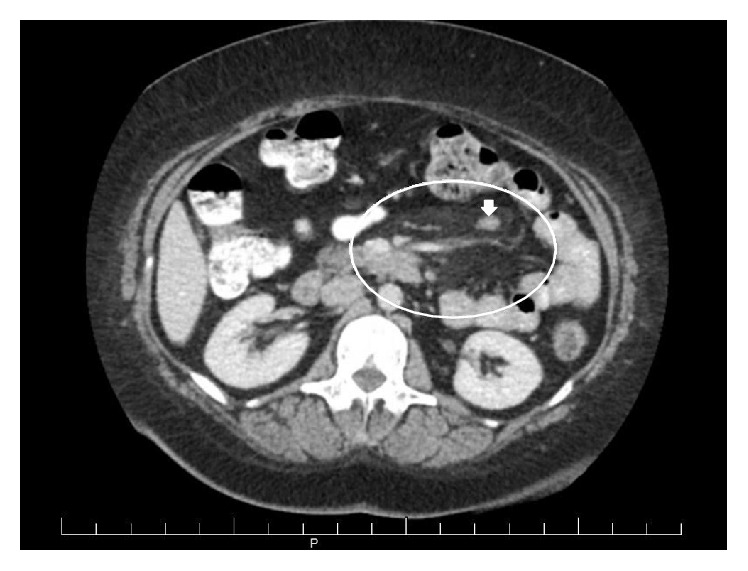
Axial CT image demonstrating mesenteric fat stranding (oval) with enlarged mesenteric lymph nodes (arrow) indicative of mesenteric panniculitis.
